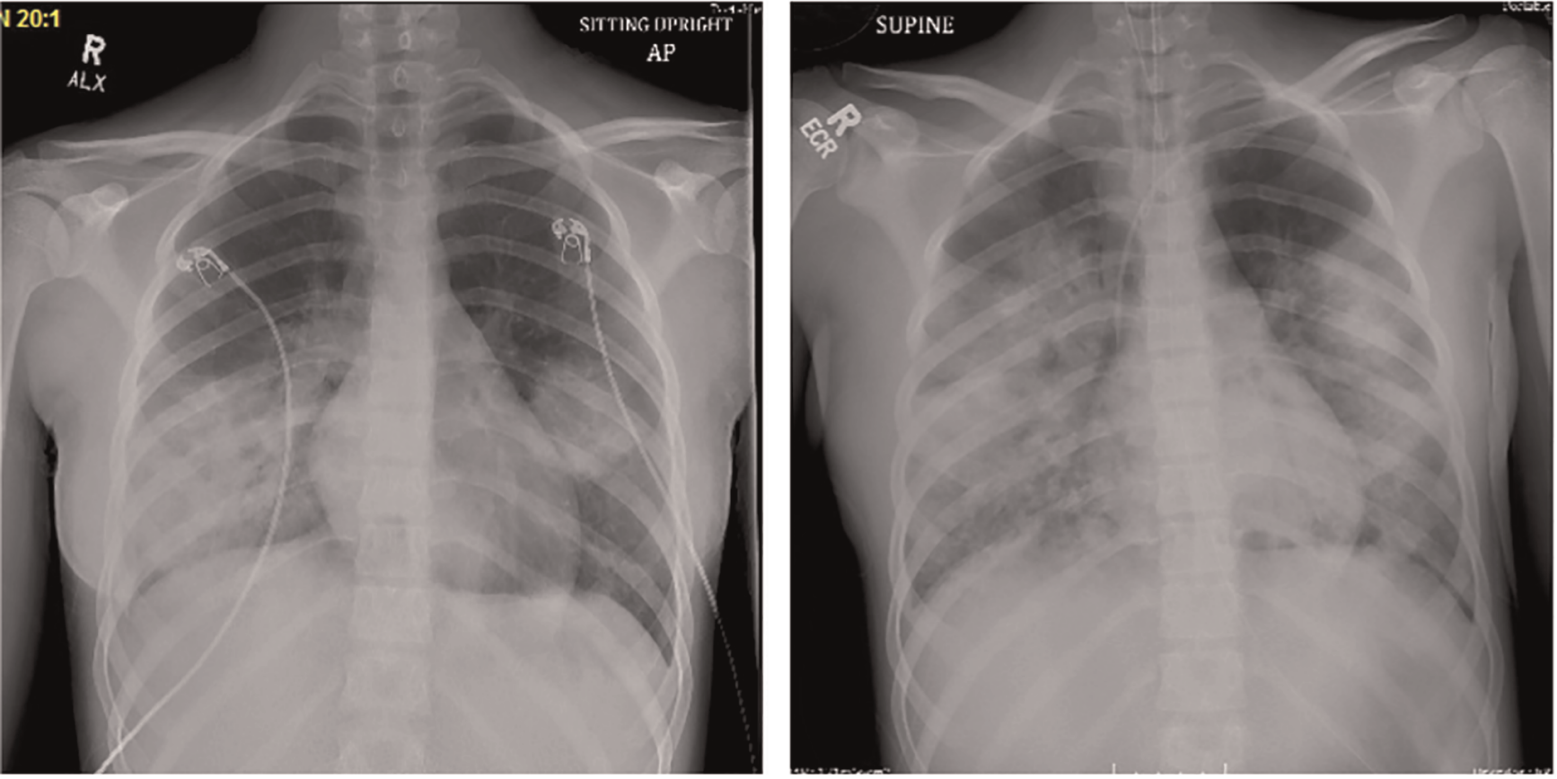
Chest radiograph on HD 1 (left). Chest radiograph on HD 4, post-intubation (right).
